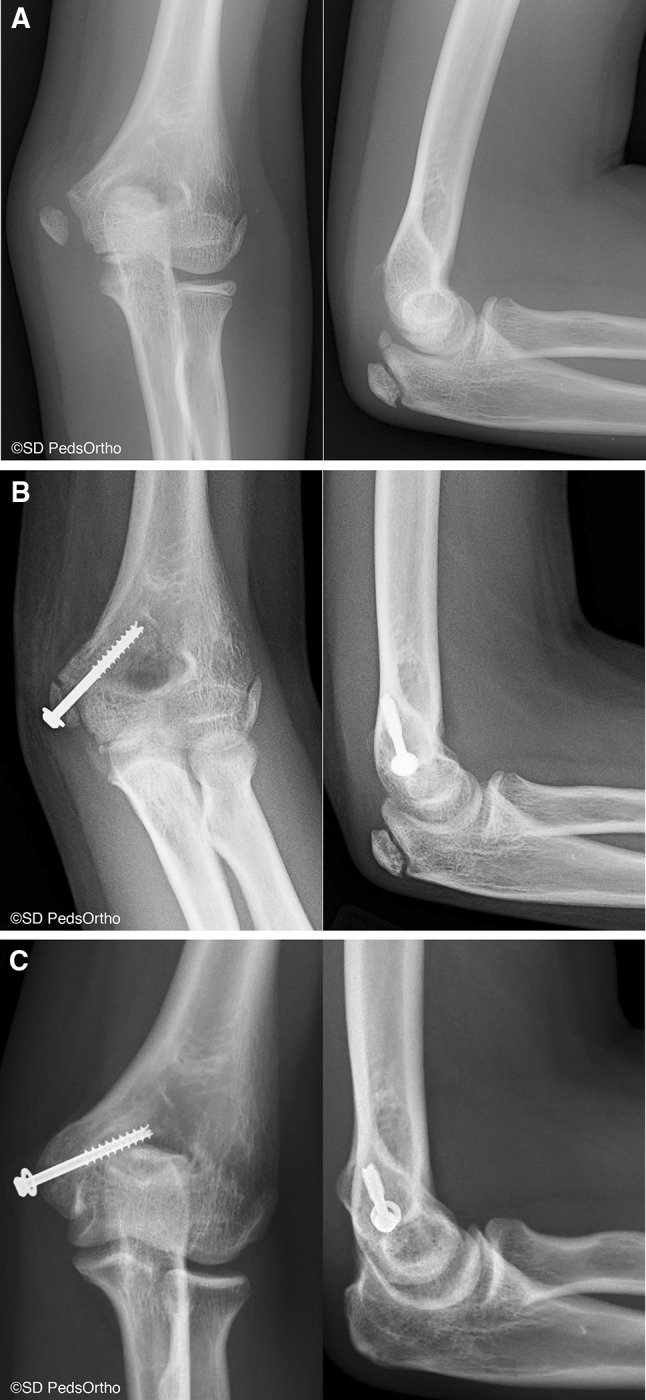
a Anteroposterior and lateral radiographs of a 12-year-old female gymnast who sustained a displaced medial epicondyle fracture. b Postoperative images. c Radiographs obtained 3.25 years postoperatively demonstrating a healed fracture with mild hardware prominence and irritation
